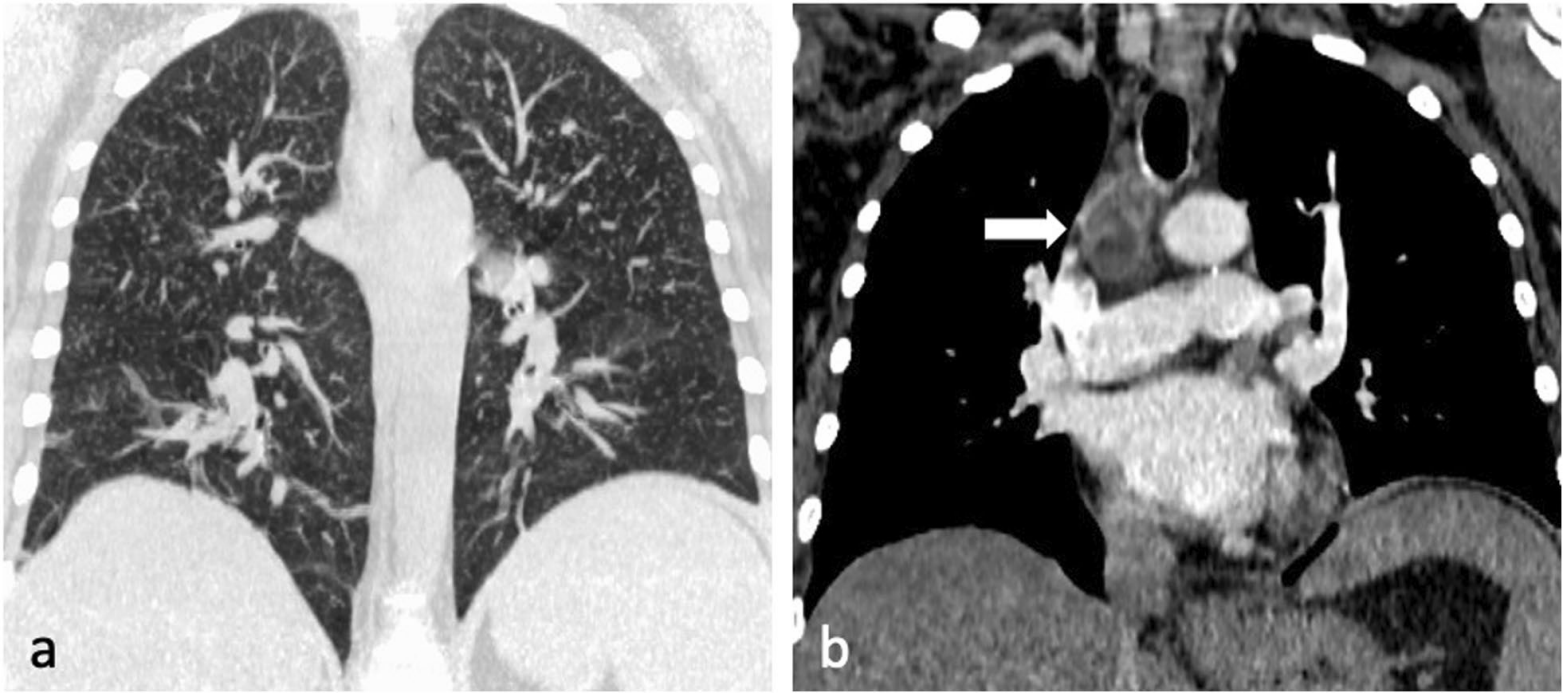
A 44-year-old female presented with symptoms of lethargy, loss of appetite, vomiting and fevers. She had been diagnosed with HIV two months prior but was not compliant with antiretroviral therapy. Her CD4 count at the time of presentation was 110 cells/mm^3^. CT demonstrates miliary nodules (lung window, coronal plane, maximum intensity projection—a) and an enlarged right paratracheal node with central low attenuation (mediastinal window, coronal plane—b, arrow) consistent with necrosis
